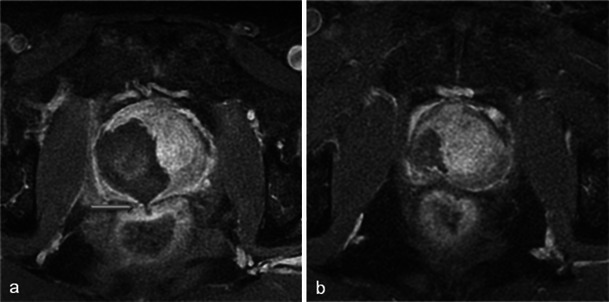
Anterior rectal wall. Right focal treatment for adenocarcinoma in a 65-year-old patient. Day-7 post-treatment (a) and 6-month post-treatment (b) axial T1 FS contrast-enhanced MR images show the presence of a thin line of necrosis extending to the anterior rectal wall (*arrow*), limited to the muscularis propria. Note the complete recovery of the rectal wall at 6 months. Also no fistulae were clinically identified
